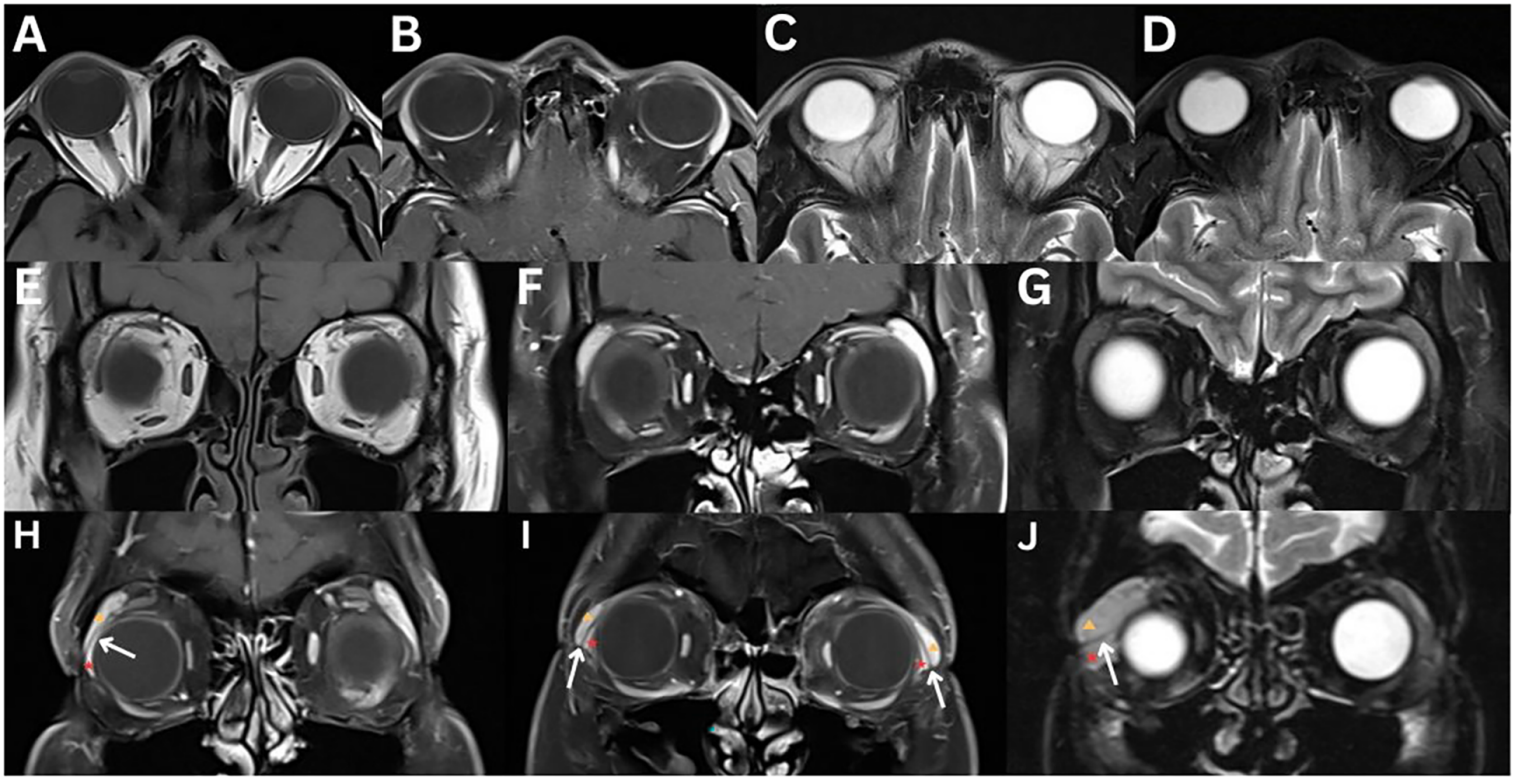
Normal MRI of the lacrimal glands (A–G). T1-weighted axial (A) and coronal (E), T1-weighted fat-suppressed contrast-enhanced axial (B) and coronal (F), T2-weighted axial (C), and T2-weighted fat-suppressed axial (D) and coronal (G) images demonstrate a lacrimal gland that is homogeneous in appearance, isointense to the surrounding extraocular muscle, in both T1- and T2-weighted images, and enhances bilaterally. Varying aetiologies of the lacrimal gland (H–J). T1-weighted fat-suppressed contrast-enhanced coronal (H, I) and T2-weighted fat-suppressed coronal (J) sequences demonstrate the lateral horn of the aponeurosis of the levator palpebrae superioris muscle (white arrow), which separates the lacrimal gland into the orbital lobe (yellow triangle) and the palpebral lobe (red star).
